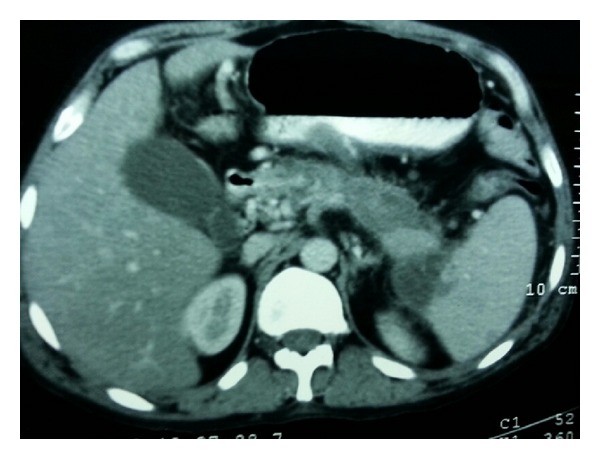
CT scan of the abdomen showing a pancreatic tail mass.
